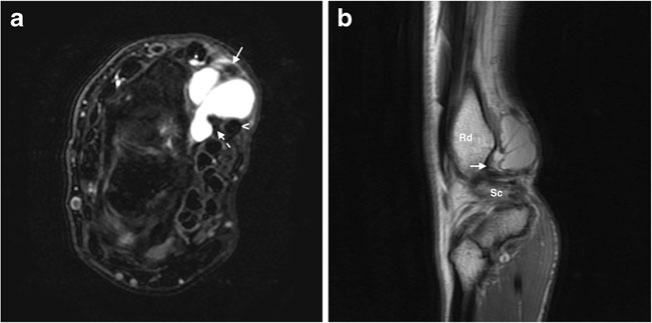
a, b. Ganglion cyst in the volar aspect of the wrist in a 55-year-old woman presenting with pain during volar flexion and paresthesia. Axial FS PD-weighted MRI (a) shows a smooth, multiloculated, homogeneously hyperintense lesion located deep and medial to the radial artery (arrow) and lateral to the flexor pollicis longus (dashed arrow) and the flexor carpi radialis (arrowhead) tendons. A tiny amount of fluid within the abductor pollicis longus and extensor pollicis brevis tendinous sheath (asterisk) is also present. The site of origin of the cyst is more evident on the sagittal T2-weighted MRI (b), which seems to originate more distally in the radioscaphoid interval (arrow). Rd, radius; Sc, scaphoid. *Case courtesy of Dr. Carlos Teiga. Radiology Department, Centro Hospitalar de Lisboa Central. Lisbon, Portugal*
